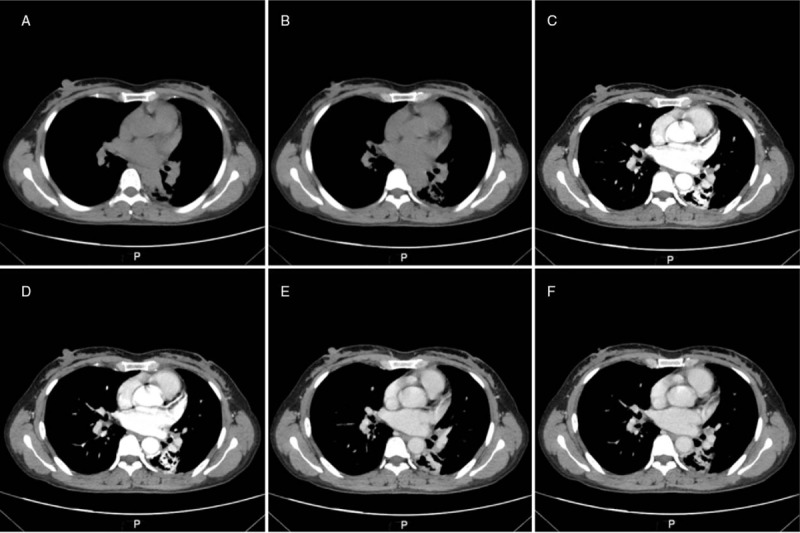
Enhanced chest CT images revealed (A–B) an enlarged lumen of the left dorsal bronchus, which was larger than the accompanying pulmonary artery as well as thick, straight, and uniform walls of the bronchi with the “signet ring” appearance; and (C–F) an enhanced “bronchi wall” in the arterial phase with decreased intensity in the venous phase.
